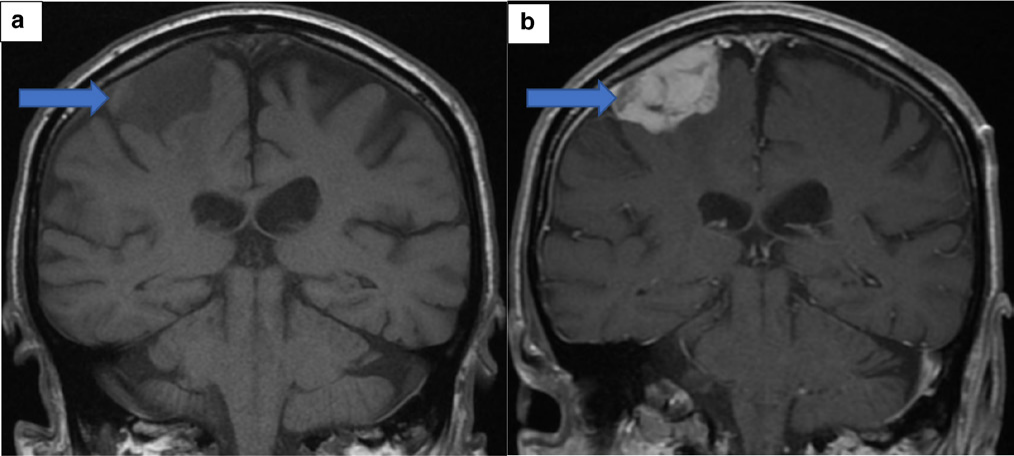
(A) Pre-Contrast Coronal T1 MRI Brain. (B) Post-Contrast Coronal T1 MRI. These images show a small focus of high T1 signal within at the right lateral aspect of the mass. This does not enhance, but demonstrates susceptibility artefact. This corresponded to the high density seen on CT. This represented the area of metastatic deposit within the meningioma
